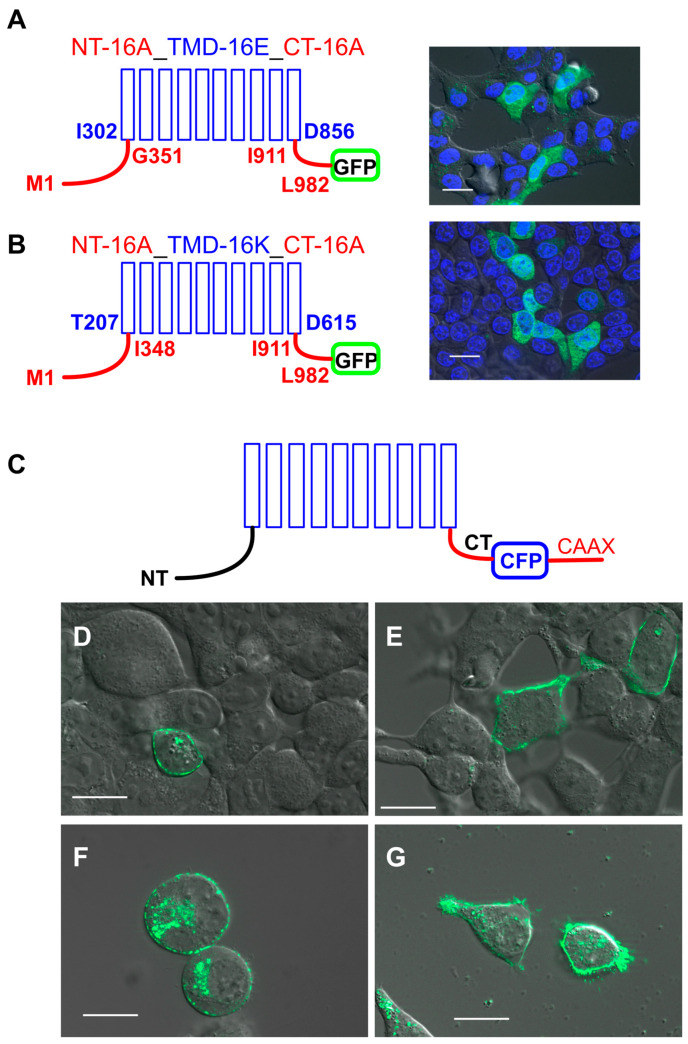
Expression of TMEM16 proteins. Chimeras of TMEM16A/TMEM16E and TMEM16A/TMEM16K were located in intracellular compartments (A,B), while TMEM16 paralogs were targeted by the CAAX motif to the plasma membrane (C-G). N-terminus (NT, amino acid (aa) M1-G351 or M1-I348) and C-terminus (CT, aa I911-L982) of TMEM16A (red lines) were cloned to the transmembrane domains (blue columns) of (A) TMEM16E (aa I302-D856) and (B) TMEM16K (aa T207-D615). In living HEK293 cells, localization of the chimeras was visualized by C-terminal-tagged GFP proteins (green). (C) Cyan fluorescent protein (CFP) (blue) and the CAAX motif (red) were cloned to the C-terminus (CT) of TMEM16 proteins. In living cells (D) TMEM16E-CFP-CAAX, (E) TMEM16H-CFP-CAAX, (F) TMEM16J-CFP-CAAX and (G) TMEM16K-CFP-CAAX were located at the plasma membrane. N-terminus (NT), Bar 20 µm.
